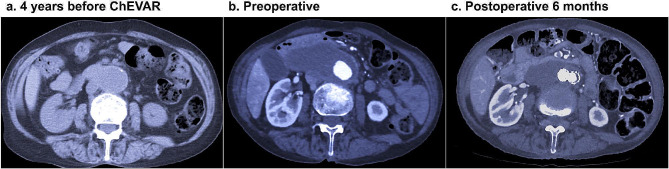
Change in size of the saccular JRAAA from 4 years preoperatively to 6 months postoperatively. a: The axial view of JRAA at 4 years before ChEVAR, measuring 54.1 × 32.3 mm in size. b: Preoperative CT findings clarified significant enlargement of JRAAA toward the sac wall side. c: CT 6 months postoperatively demonstrates noticeable reduction of JRAAA. ChEVAR, chimney endovascular aortic repair; CT, computed tomography; JRAAA, juxtarenal abdominal aortic aneurysm
